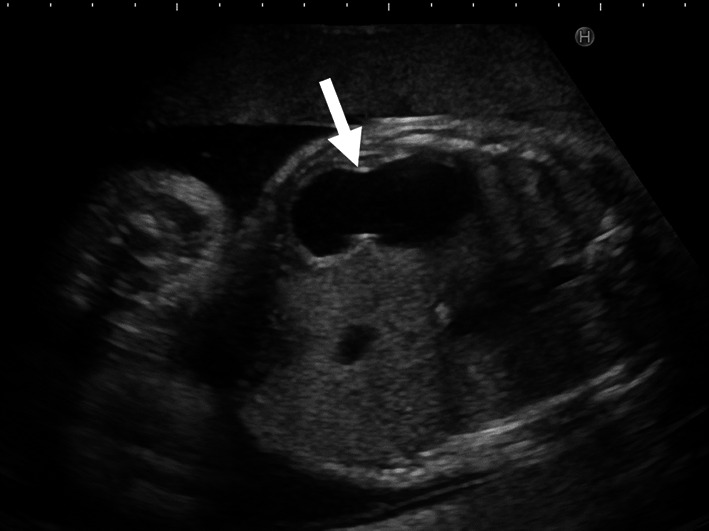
Prenatal ultrasonography revealing polyhydramnios and dilated stomach (*arrow*) (Case 1)
